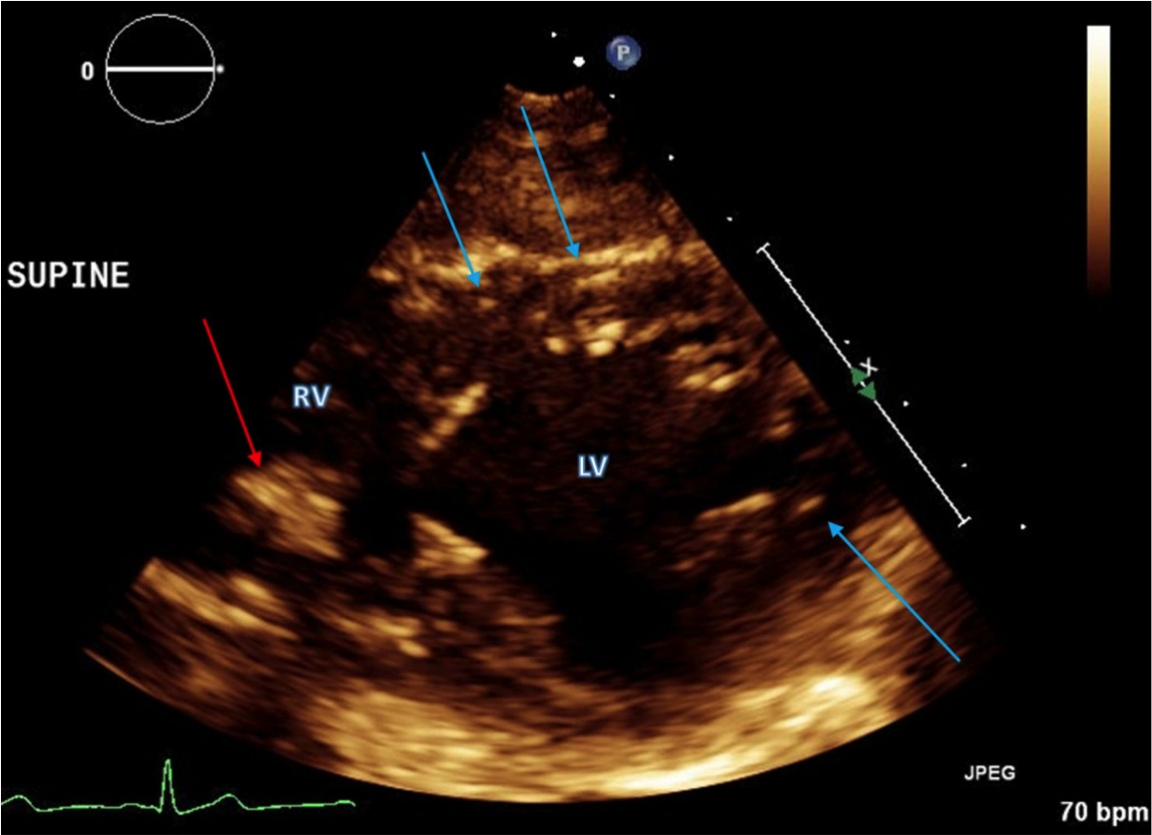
Transthoracic Echocardiogram of a Patient with Tuberous Sclerosis Complex Transthoracic echocardiogram in short axis demonstrates an echogenic mass in the midseptal region of the right ventricle (red arrow). Additional punctate echogenic foci are also identified (blue arrows). RV = right ventricle; LV = left ventricle.
